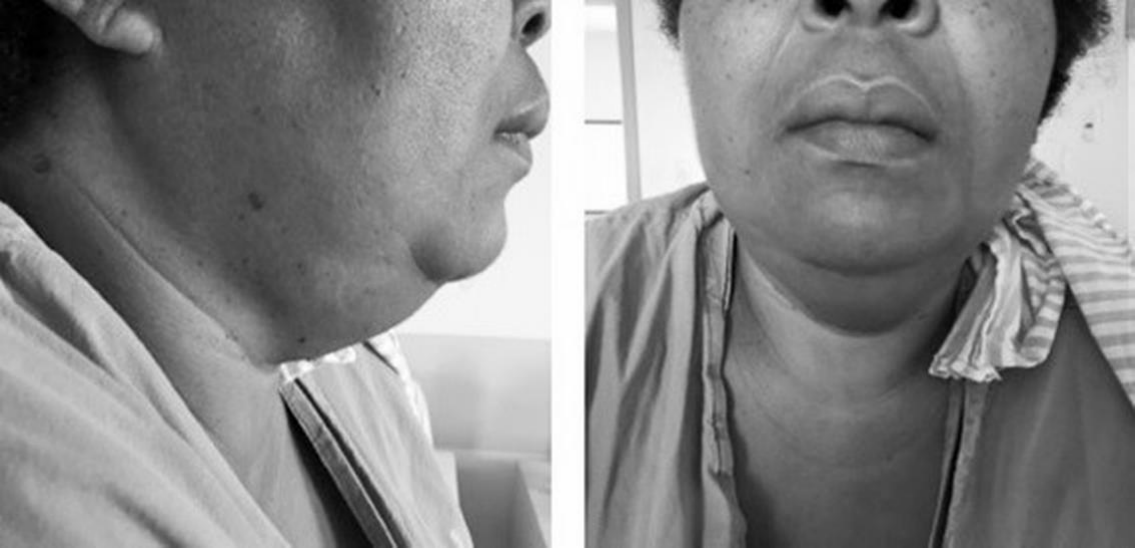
Imagens demonstrando abaulamento cervical. Ultrassonografia confirmou correspondência com aumento das glândulas parótidas. Imagens do quinto dia após exposição ao meio de contraste iodado.
